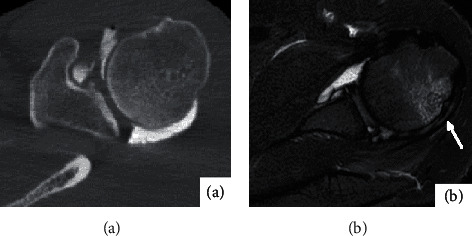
Male, 23 years old, anterior dislocation of left shoulder for 1 month. (a) shows the transverse bone window image of CTA on the left shoulder. No obvious abnormalities were found in the morphology and density of the humerus. (b) shows the PDW-FS image of the left shoulder on the horizontal axis of MRA, and the signal of the patellar bone marrow edema outside and above the humeral head was shown in the white arrow.
